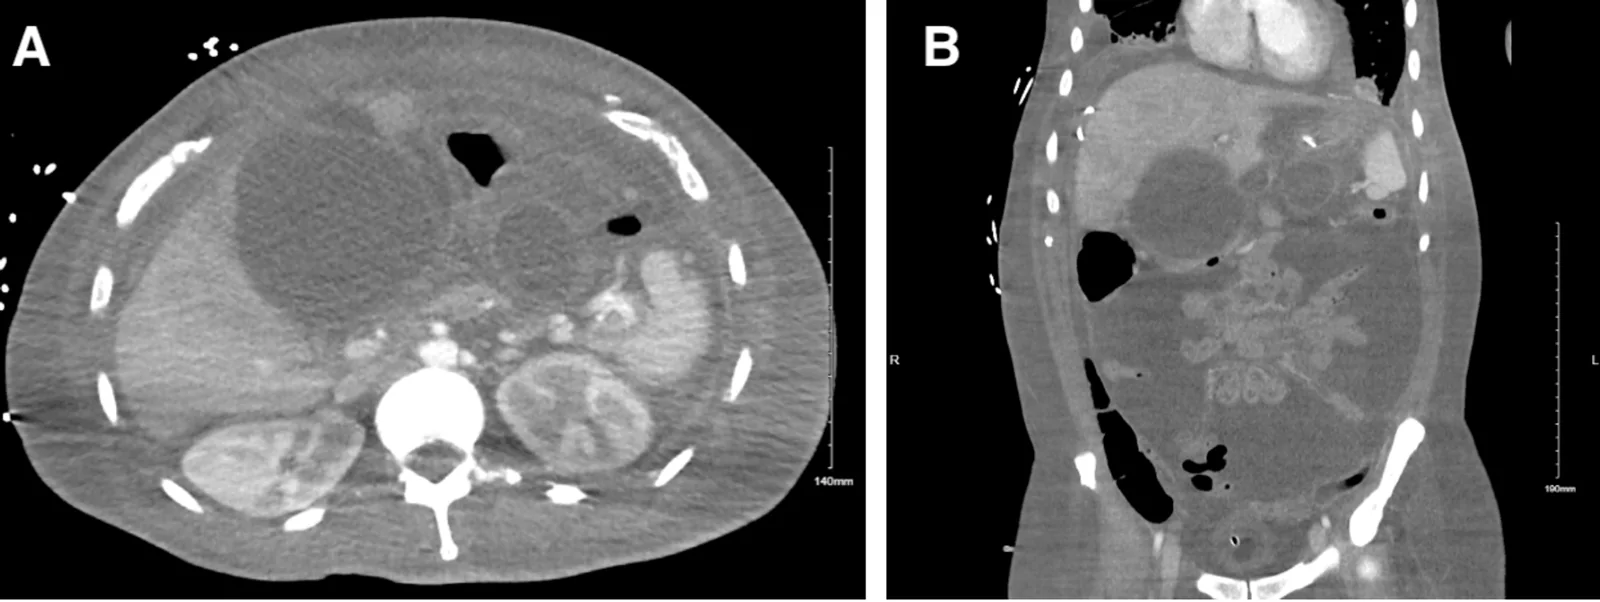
CT of the Abdomen and Pelvis Demonstrating Loculated Ascites and Peritoneal Drain Placement (A) Axial contrast-enhanced CT of the abdomen and pelvis demonstrating a large perihepatic loculated ascitic collection. (B) Coronal contrast-enhanced CT of the abdomen and pelvis showing multiple loculated intra-abdominal fluid collections, with a pigtail drainage catheter in the right upper quadrant demonstrating minimal output.
